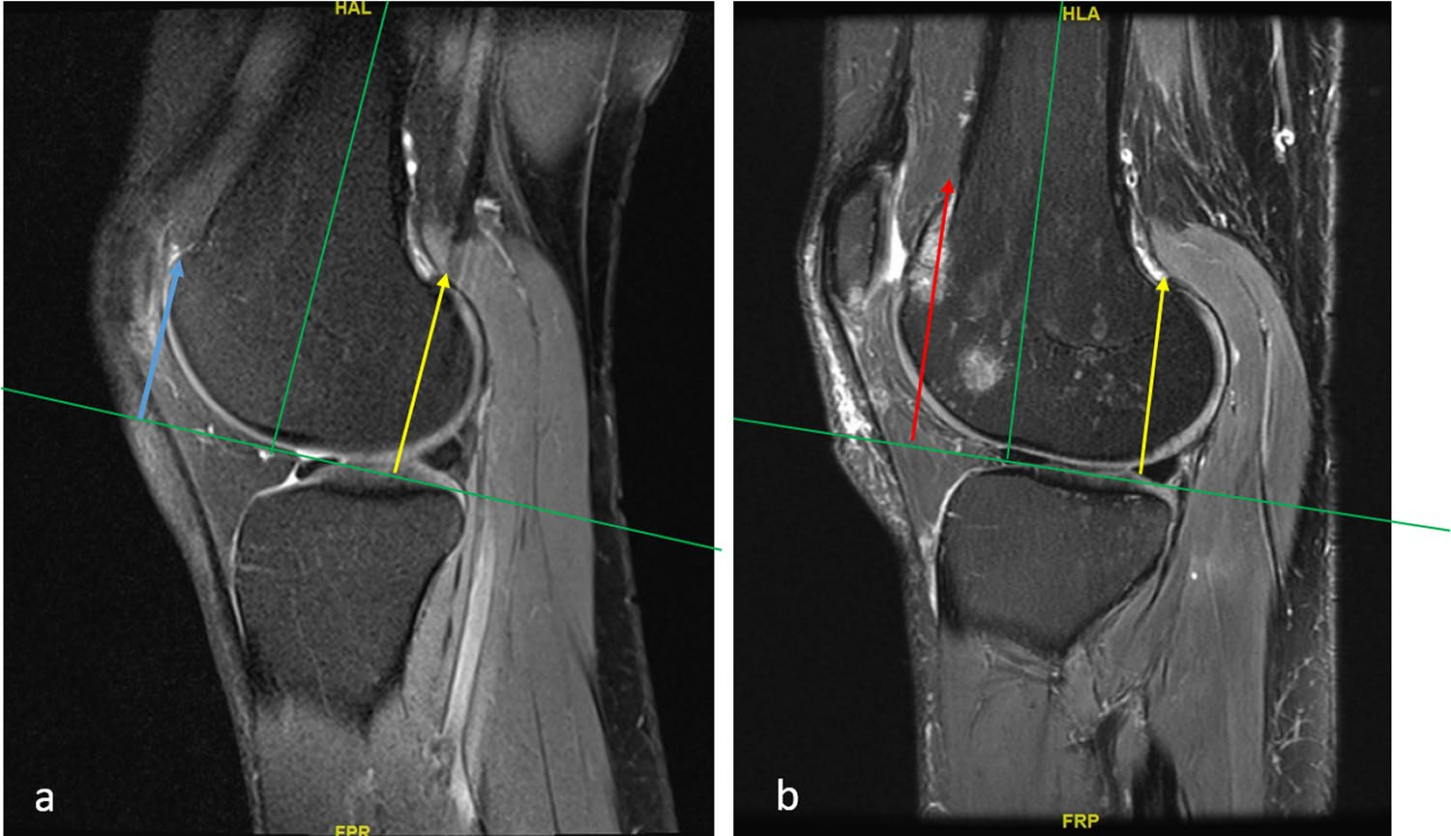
Preoperative (a) and postoperative (b) MRI images of a patient with a lateral trochlear lengthening osteotomy. Note the difference between the anterior condyle length before the operation (blue arrow, a) and after the operation (red arrow, b). The lateral condylar index was 84% preoperatively and 120% postoperatively. The yellow arrow represents the posterior condyle length and the green line the femoral axis (a, b)
